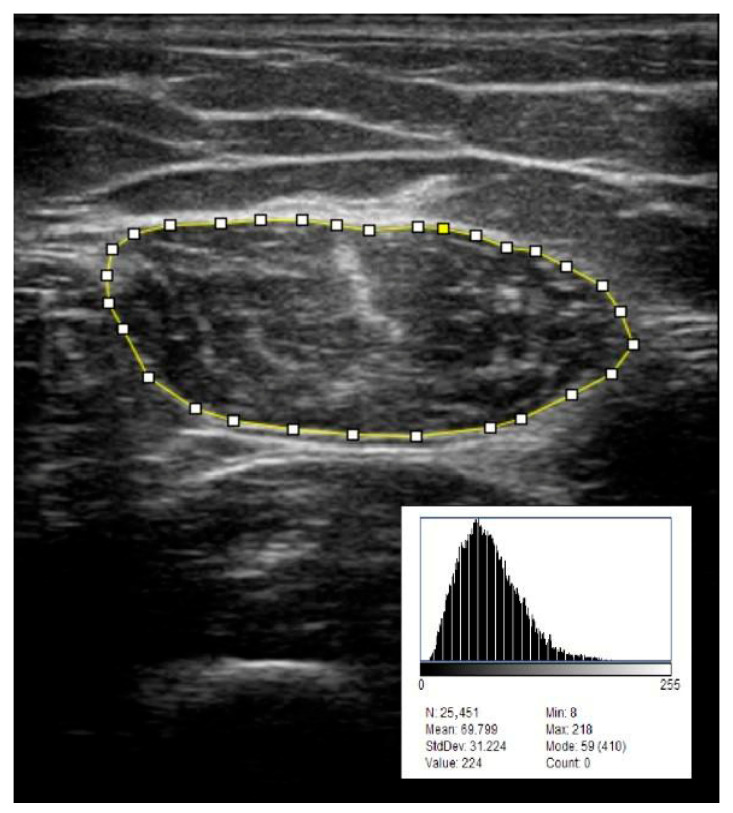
Assessment of echo intensity. The region of interest (ROI) outlined in yellow on the ultrasound image was analyzed for grayscale intensity. The histogram shows pixel values (0–255) and provides descriptive statistics including the number of pixels (N), mean, standard deviation (SD), minimum, maximum, and mode.
